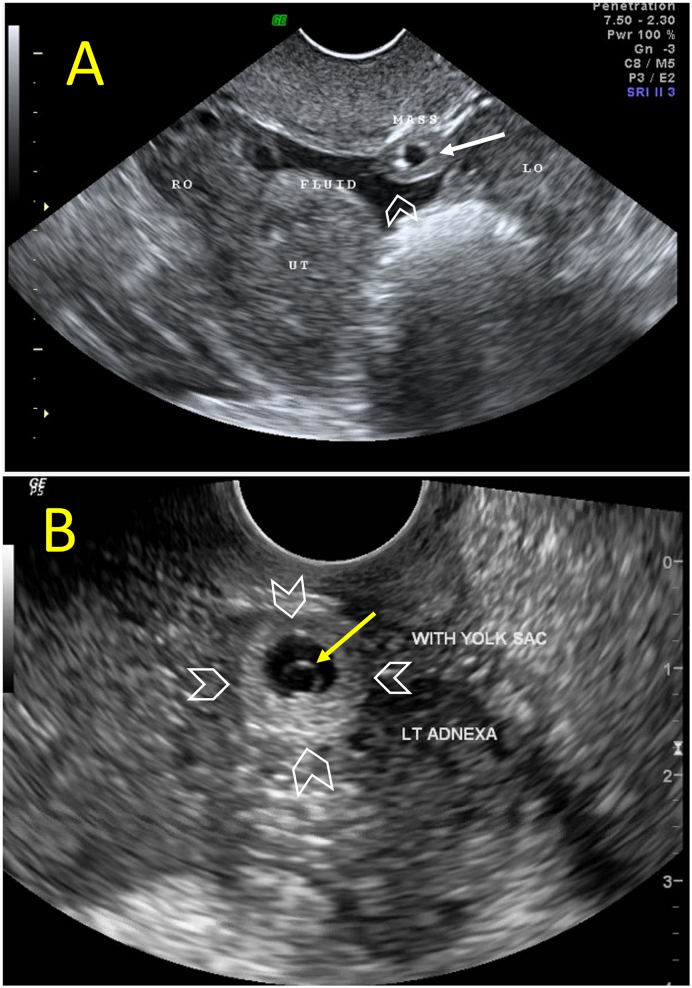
Transvaginal ultrasound scan. Transvaginal ultrasound scan revealed absence of intrauterine gestational sac. A complex mass measuring 1.2 × 0.9 × 1 cm was seen (white arrow) close to the left ovary (LO) and was suspected to be a gestational sac at the left adnexa There was minimal fluid in the pouch of Douglas (arrow head) (A). Follow up transvaginal ultrasound 4 days later showed that the mass has increased in size (1.49 × 1.19 × 1.44 cm) (arrow heads) which contained a well-defined yolk sac (yellow arrow) (B). (For interpretation of the references to colour in this figure legend, the reader is referred to the web version of this article.)
